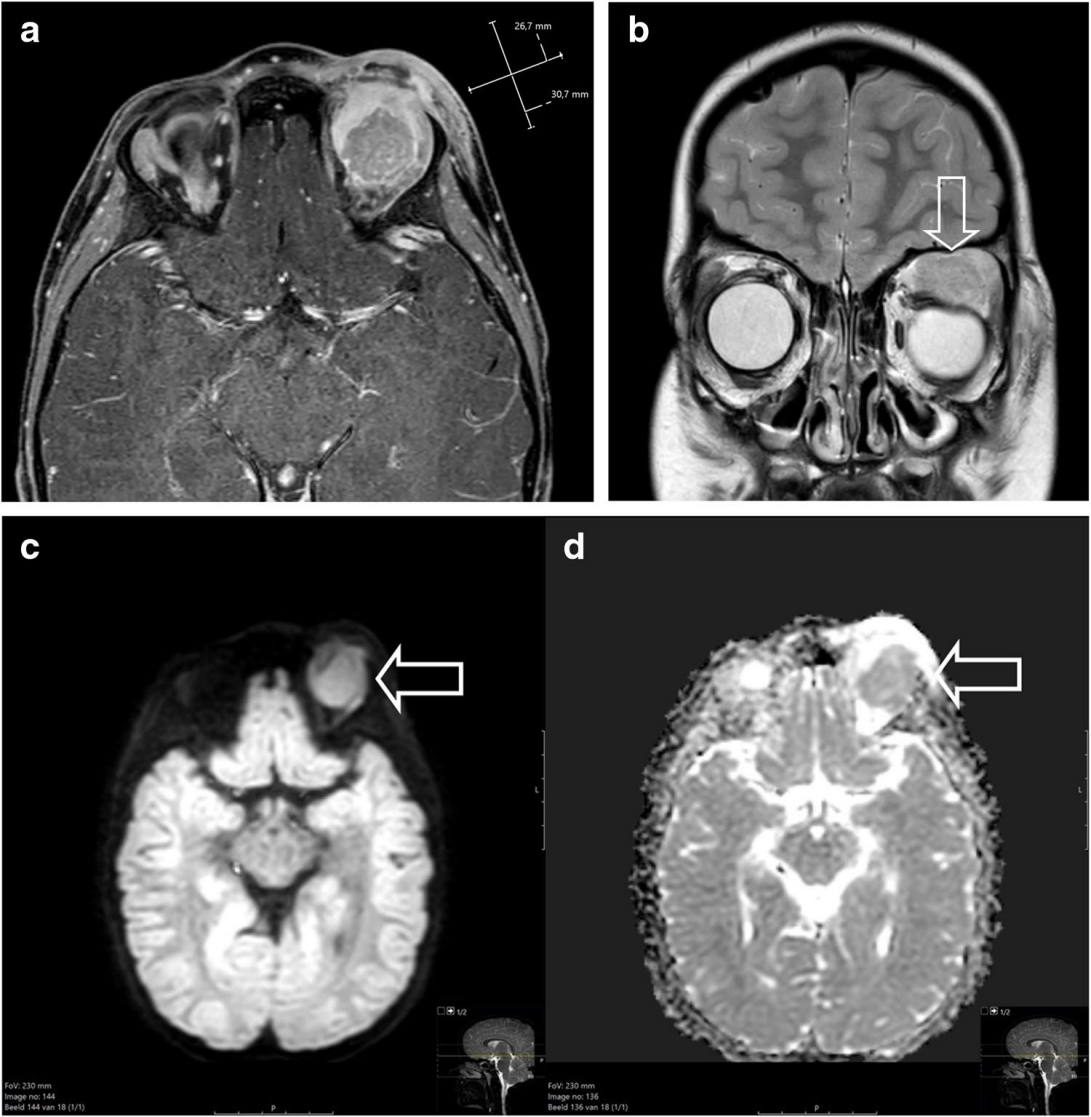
a, b Magnetic resonance image of a primary orbital tumour in a 6-year-old boy (patient 1) after surgical biopsy and confirmation of rhabdomyosarcoma. a Axial T1 after gadolinium administration with fat suppression shows a well-described mass with inhomogeneous enhancement. Post biopsy swelling and subcutaneous and cutaneous oedema are seen. b Coronal T2 turbo spin echo image shows a hyperintense signal and compression by the mass of the eye bulb (*arrow*). c, d Diffusion weighted imaging b-value 800 (c) and apparent diffusion coefficient (ADC, d) show increased diffusion restriction with hyperintense signal on the b800 and decreased signal on the ADC map (*arrows*)
